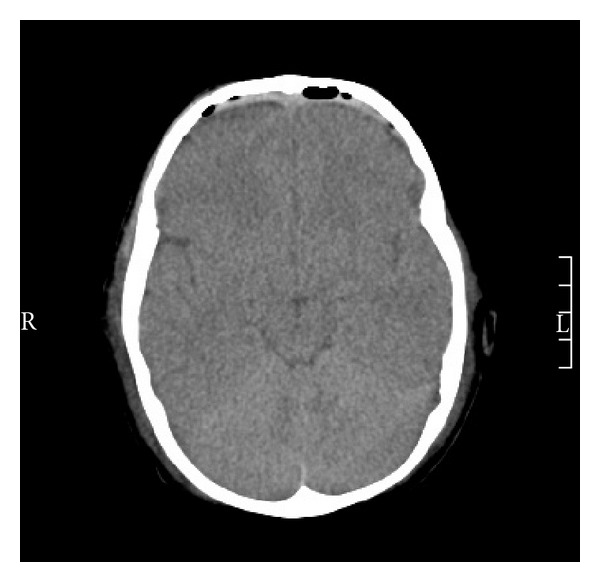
Postoperative control axial CT scan.
